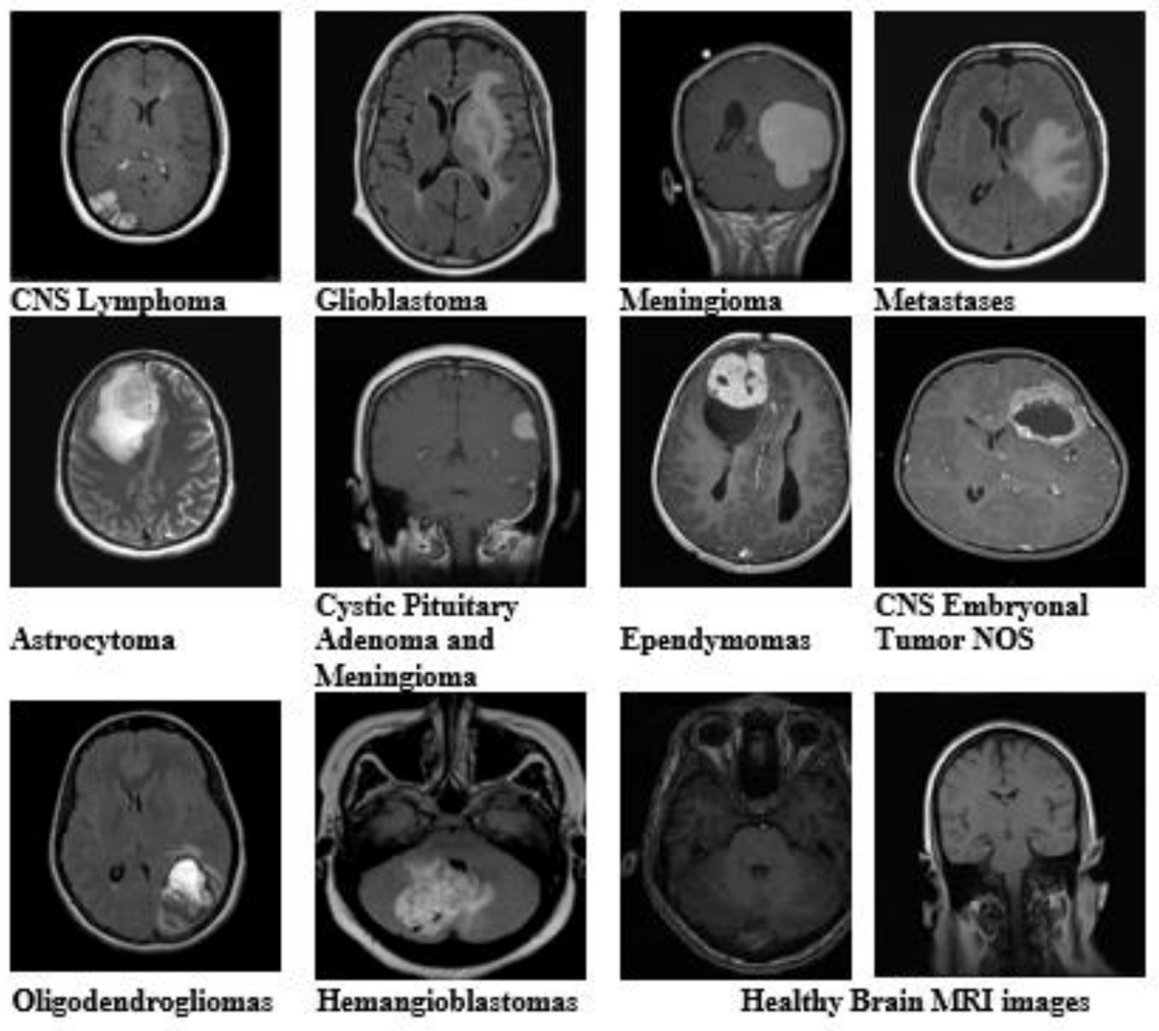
Brain MRI images of all tumor types and healthy brain MRI images from the dataset with different variants and modalities.
